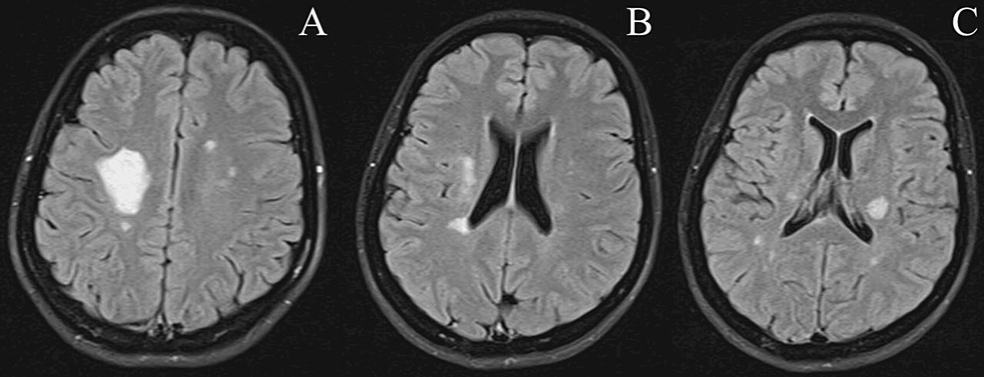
Brain MRI (A-B-C) axial FLAIR images show variable sized lesions in deep white matter of bilateral cerebral hemispheres with abnormal high signal intensity. The largest lesion in the right is 3 cm × 2.5 cm in size.
